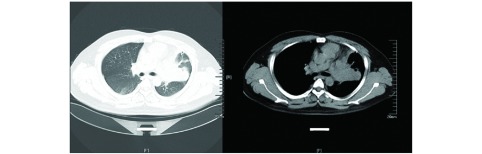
胸部CT（2016-11-12）：左肺门软组织影，大小约7.8 cm×8.8 cm，分叶状，密度尚均匀，周围可见肿大淋巴结影。 Chest CT scan (2016-11-12): A lobulated soft tissue giant tumor located in the left pulmonary hilum with the size of about 7.8 cm×8.8 cm showed homogeneous density; lymphadenopathy around it can be seen. CT: computed tomography.
